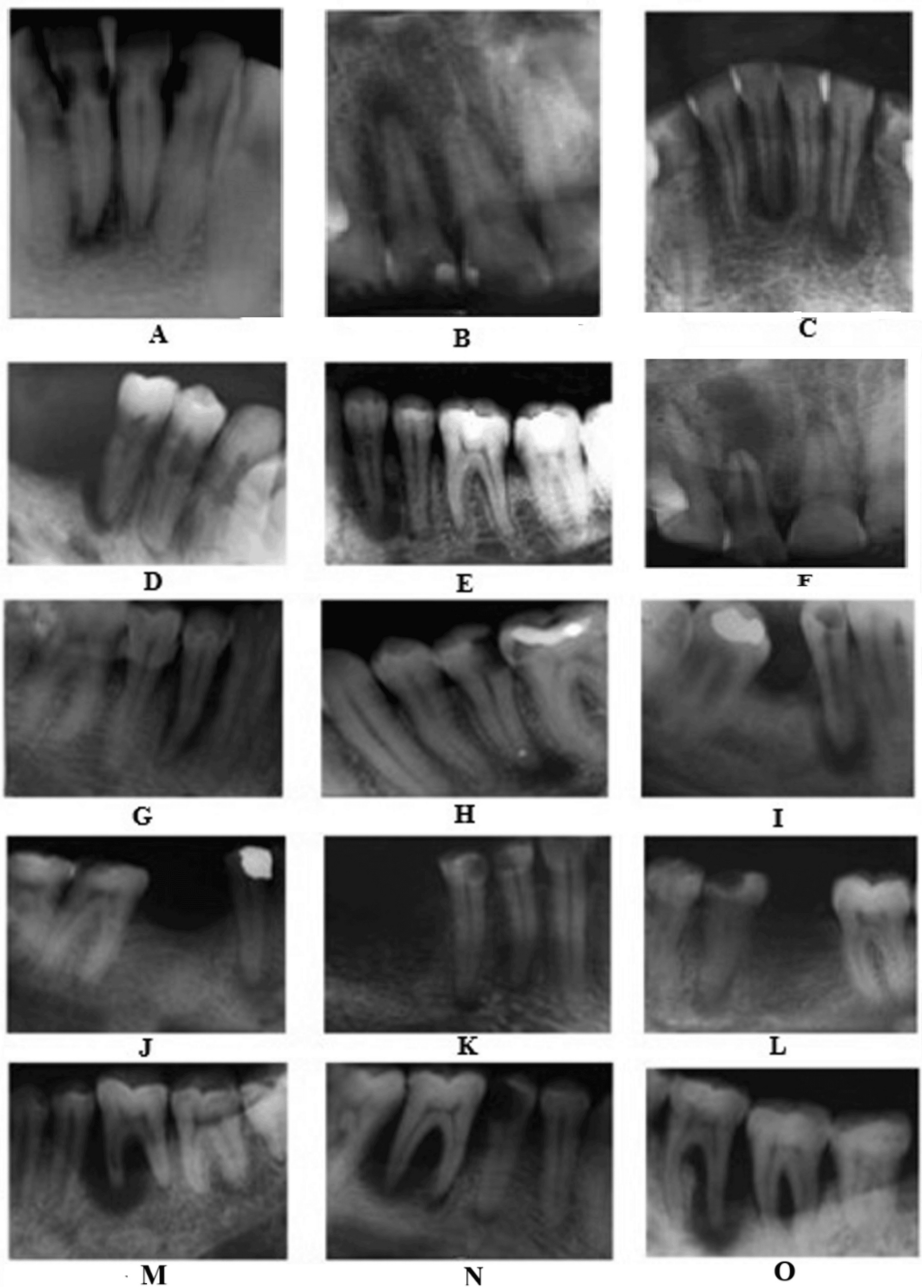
Periapical radiographs of patients positive to P. gingivalis with endodontic-periodontal lesions (A) Periapical lesion 31, 41. (B) Periapical lesion 11, 12. (C) Periapical lesion 41, 32. (D) Periapical lesion and bone loss 45. (E) Periapical lesion 34 and periodontal space widening with periapical changes 35. (F) Large periapical lesion 12. (G) Periapical lesion and vertical bone loss 44. (H) Caries and periapical lesion 35. (I) Periapical lesion and bone loss 45. (J) Coronal radiopacity and periapical lesion 44. (K) Caries and periapical lesions 44, 45. (L) Caries and periapical lesion 35. (M) Large periapical lesion with severe bone loss 36. (N) Large periapical lesion with severe bone loss 46. (O) Large periapical lesion with severe bone loss 36, 37.
